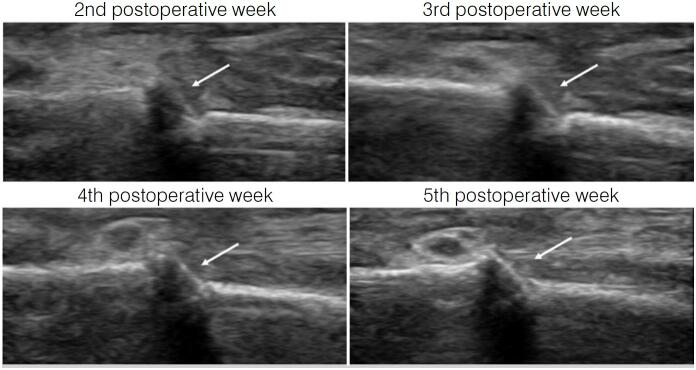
Serial postoperative ultrasonographic images demonstrating bone callus formation. At the 5th postoperative week, Kirschner wires were removed after visualization of the bone bridge at the fracture site.
